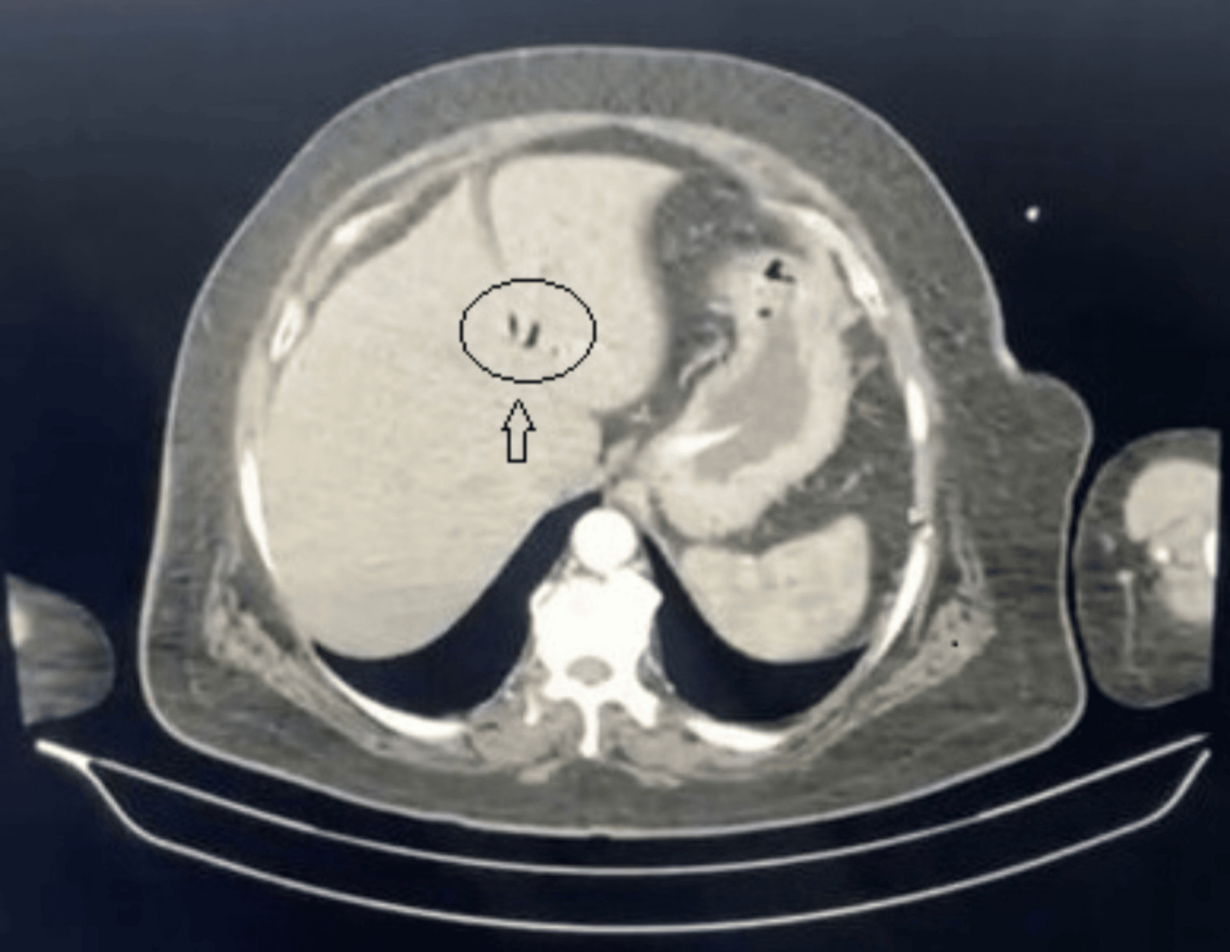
Axial computed tomography (CT) scan of the abdomen showing air within the biliary tree (pneumobilia, arrow).
